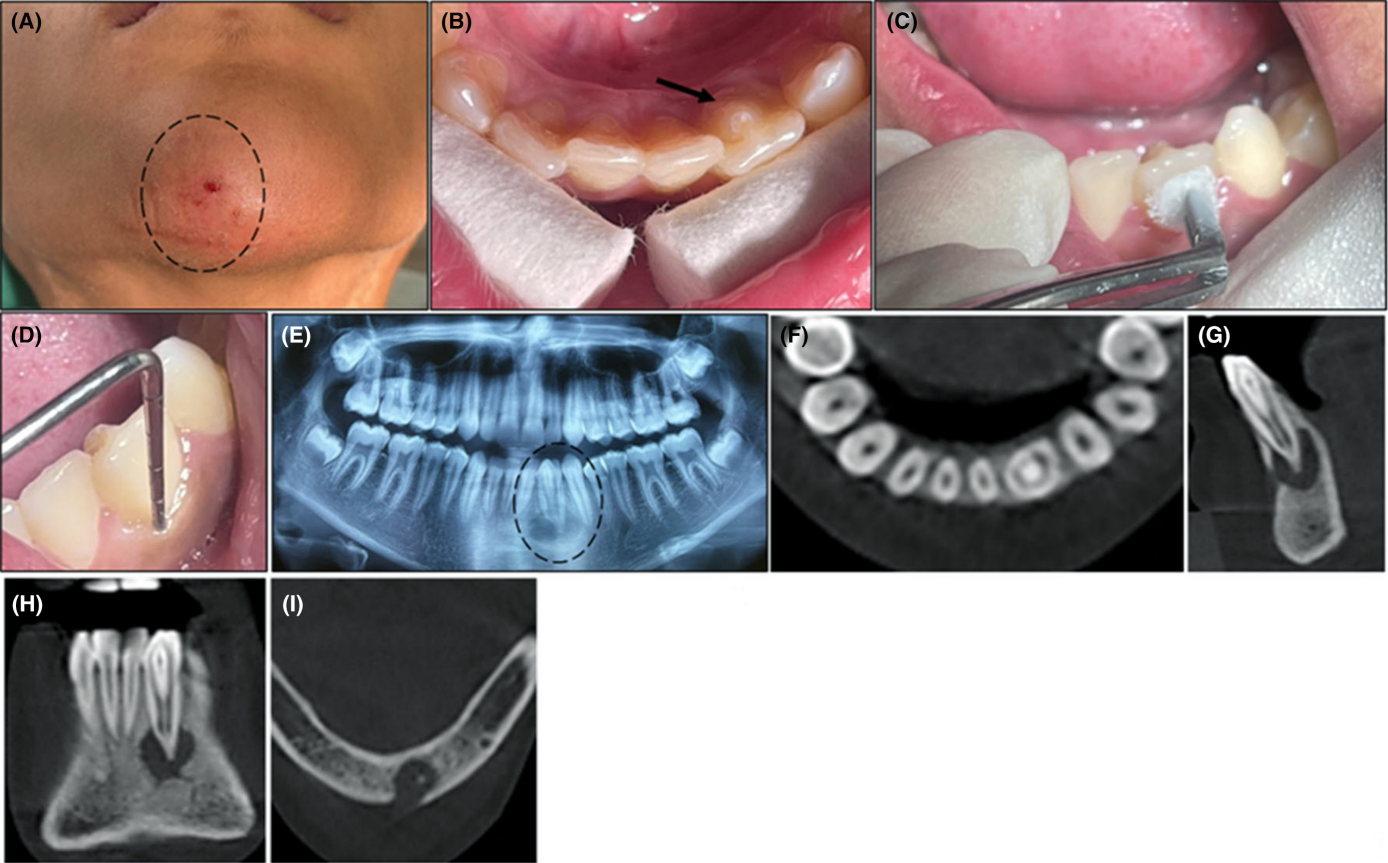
(A) An extra oral fistula in right side of the chin. (B) Talon cusp. (C) Cold sensibility test revealed tooth necrosis. (D) Probing depth was in normal limited. (E) Panoramic radiograph shown a large periapical lesion in left mandibular lateral incisor affected by dens invagination anomaly. (F) CBCT, axial view. (G) CBCT, coronal view. (H) CBCT, sagittal view. (I) CBCT, axial view demonstrated cortical bone perforation.
